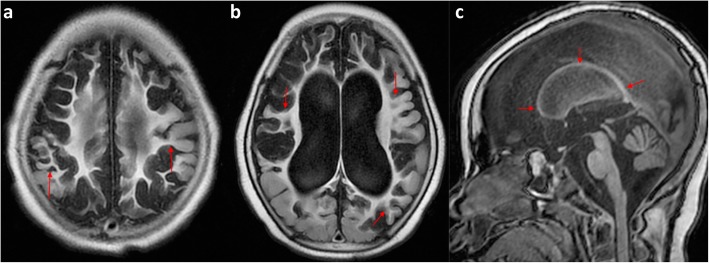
a–c MRI imaging in a 5-year-old girl who sustained a severe partial-prolonged hypoxic-ischaemic injury at term gestation. The sequential FLAIR images from the vertex (a) to the level of the lateral ventricle bodies (b) demonstrates severe volume loss of both cortex and subcortical white matter extending to involve the deep white matter of the entire cerebral hemisphere bilaterally, with only a strip of periventricular white matter remaining and showing abnormal high signal throughout. There is also prominent ulegyria (arrows) of the watershed continuum from anterior, over the vertex, through the peri-Sylvian region, and into the posterior watershed. The sagittal midline T1-weighted image (c) demonstrates corresponding corpus callosum thinning throughout its length (arrows)
